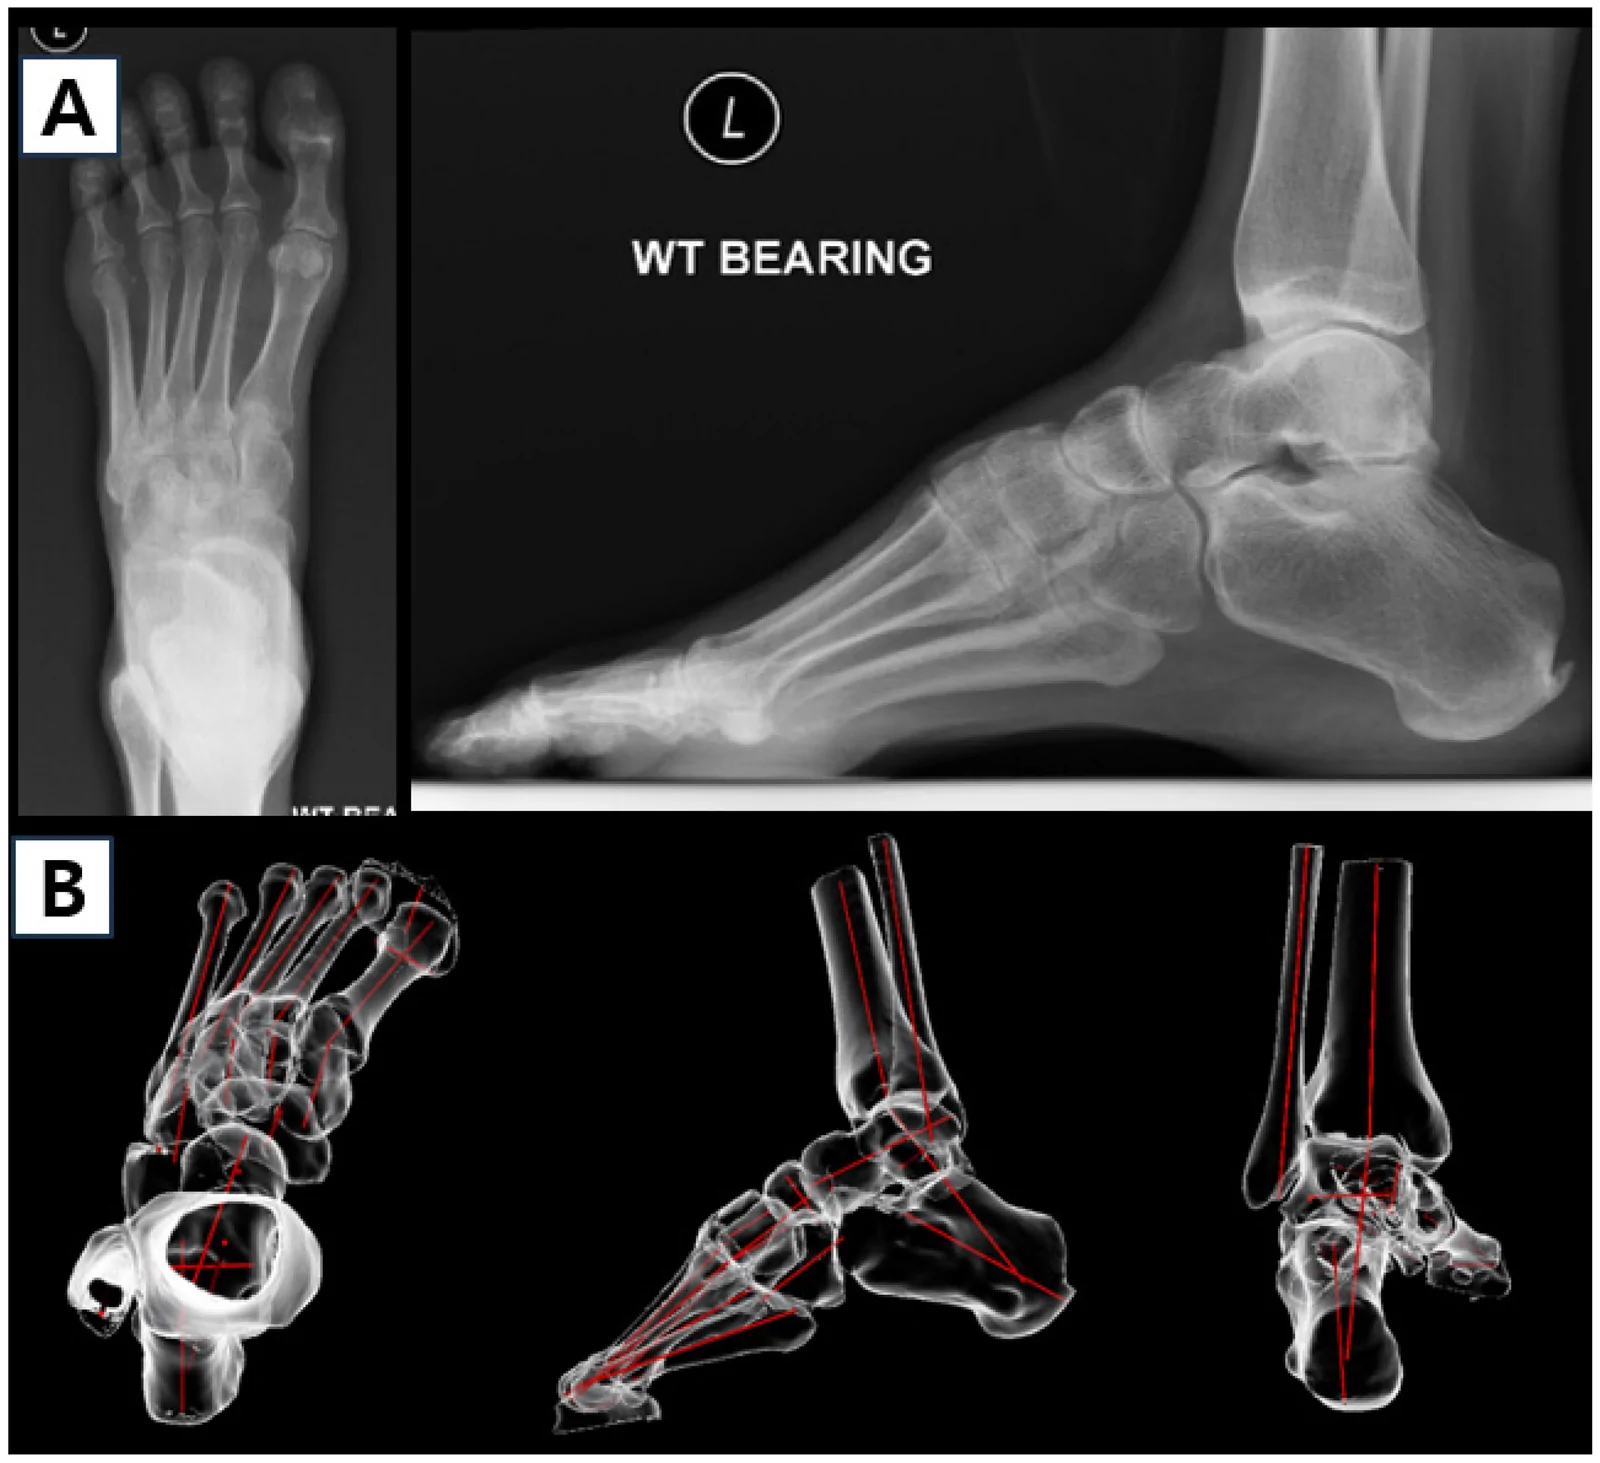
Images of CMT foot deformity. (A) Standing anteroposterior and lateral foot radiographs. (B) Segmental bone axes (red lines) identified using three-dimensional automated analysis software on weight-bearing CT images.
